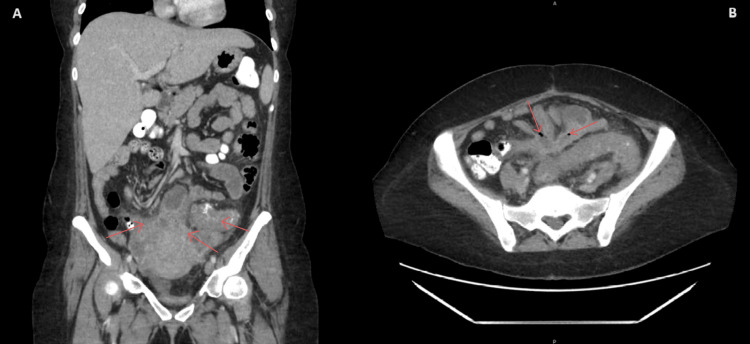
Abdominal and Pelvic CT with IV Contrast Imaging before Lower Anterior Resection Impression: A: Severe diffuse sigmoid colon wall thickening which could reflect colitis or diverticulitis, hyperdensity extrinsic to the sigmoid colon. Pelvic small bowel inflammatory changes with wall thickening, tethering, and areas of distension. B: Small pelvic air-fluid collection suspicious for an interloop abscess on axial image on the right. Diverticulitis may be classified as Hinchey/Kaiser Ib according to CT imaging impression.
